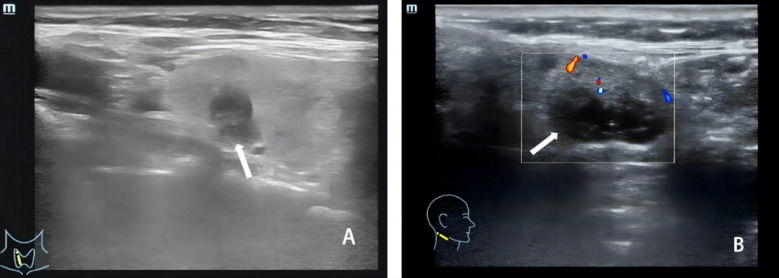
Ultrasound examination of lymph node metastasis in thyroid malignant tumors. A: The tumor has a small volume and no lymph node metastasis is observed in the neck; B: The tumor has a large volume, with abnormal lymph node metastasis visible in the neck. The normal lymph node structure disappears, and the boundary between the cortex and medulla is unclear. Calcification can be detected in the cortex of lymph nodes, and blood flow is disordered.
